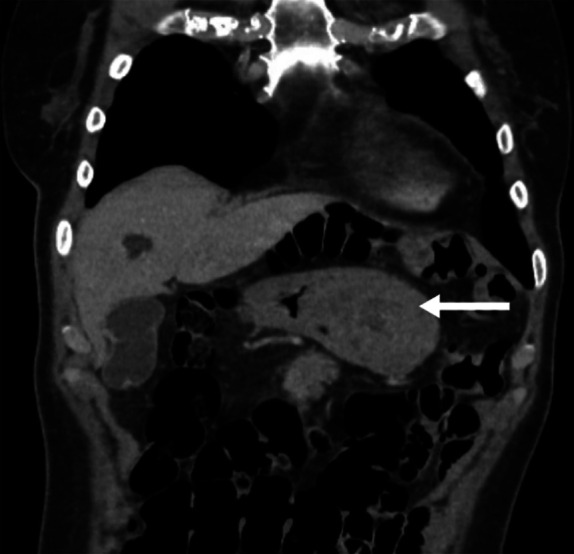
Computed tomography of the abdomen/pelvis demonstrated 6-cm fundic mass invaginating into the antrum (arrow).
